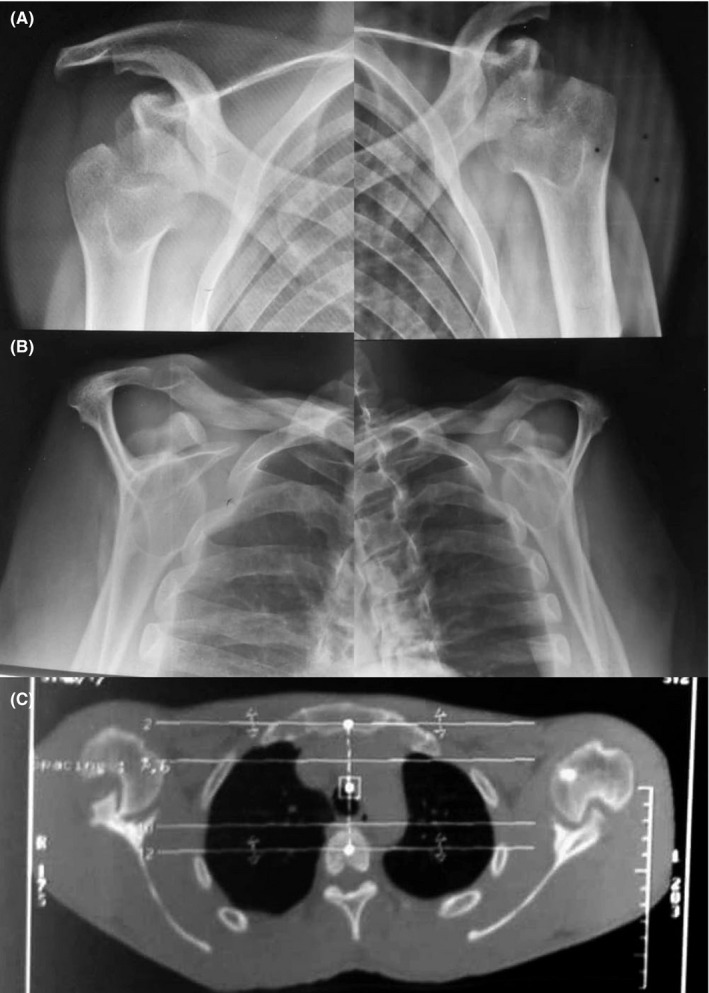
Shoulder radiographs and CT scan (Case 2). Between inferior glenoid rims and posterior Hill‐Sachs lesion (arrows) are arranged neoglenoid joints
